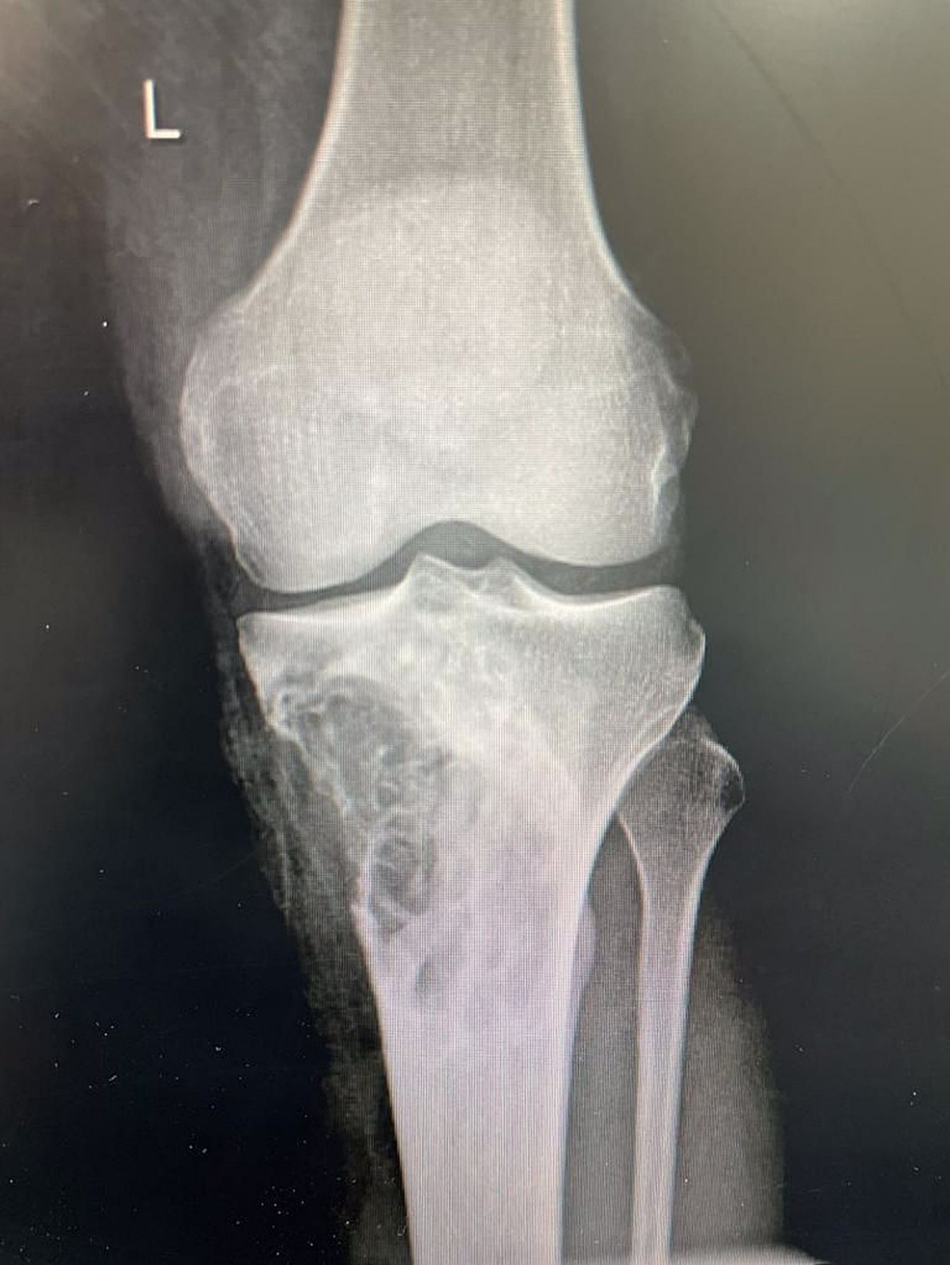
Pre-operative x-rays showing mix osteolytic osteosclerotic lesion involving the proximal tibial metaphysioepiphyseal with a narrow zone of transition and multiple lobulations, also a cortical breakthrough.
